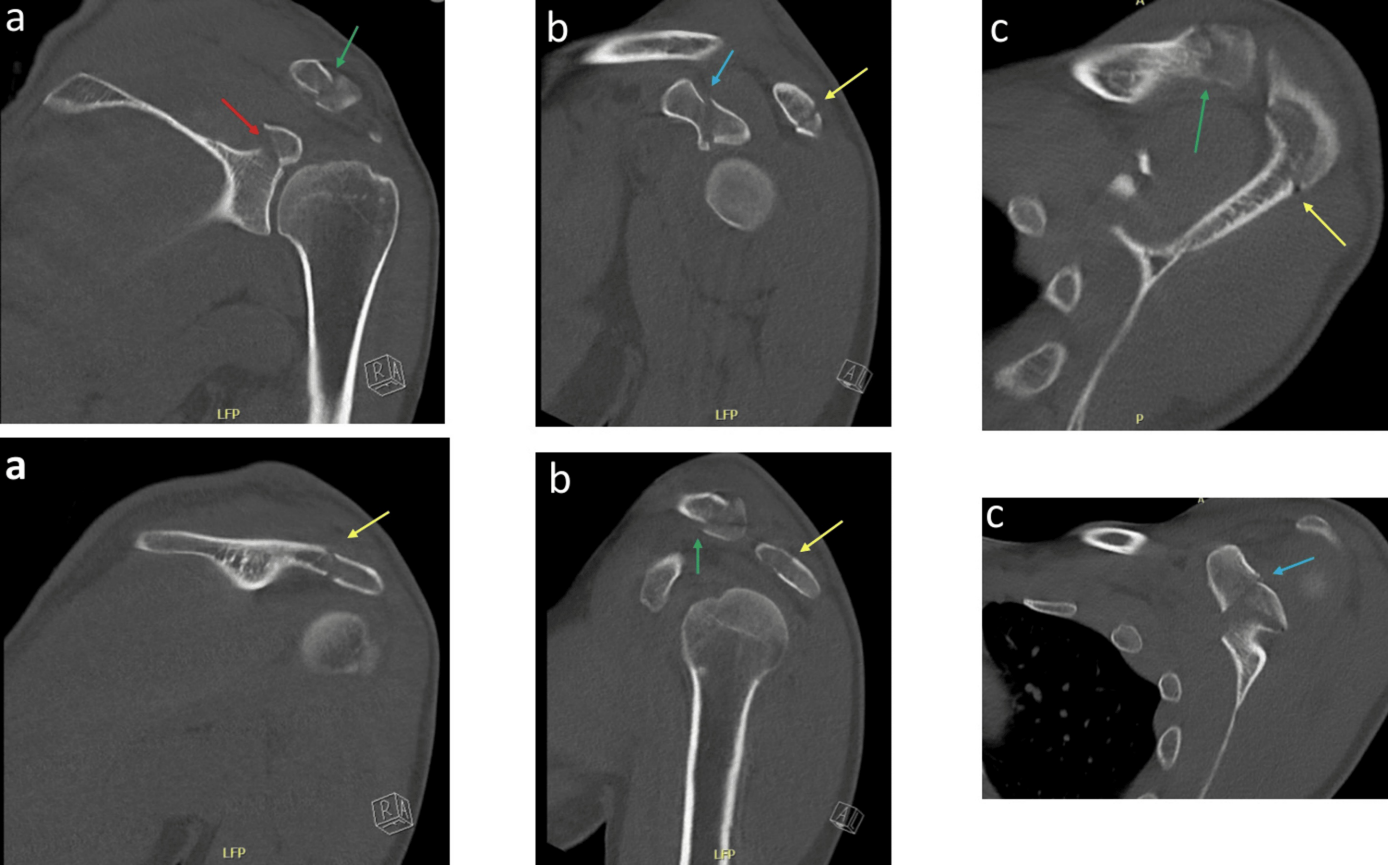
Preoperative computed tomography (CT) images of the shoulder girdle Preoperative CT images in the coronal (a), sagittal (b), and axial (c) planes. The arrows indicate fractures of the glenoid (red), acromion (yellow), distal clavicle (green), and coracoid (blue).
